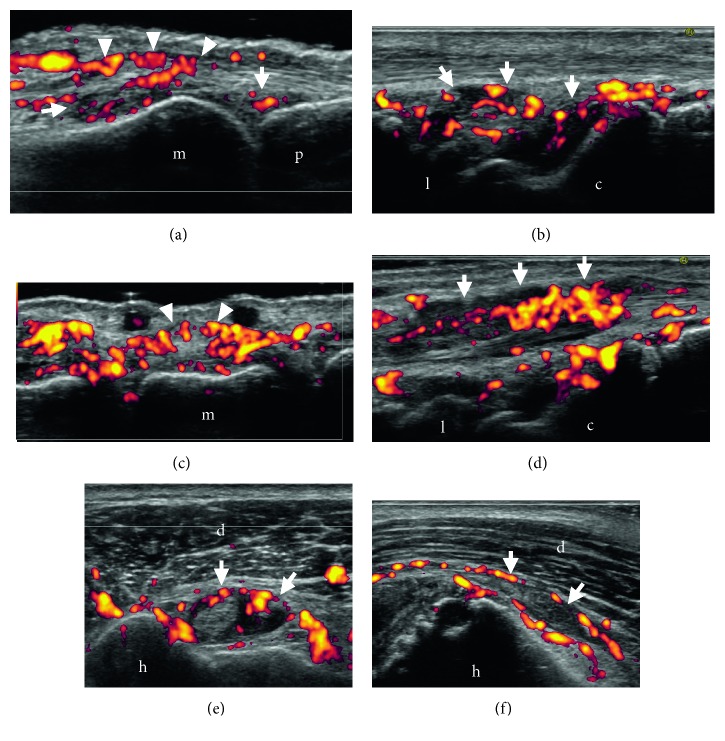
Severe synovitis detected by ultrasonography at 3 months after the introduction of nivolumab. (a) Articular synovitis (arrow) and peritendinitis of digital extensor tendon (arrowhead) in the 2nd metacarpophalangeal (MCP) joint (dorsal aspect, longitudinal view). (b) Articular synovitis (arrow) in a radiocarpal-intercarpal joint (dorsal aspect, longitudinal view). (c) Peritendinitis of digital extensor tendon (arrowhead) in MCP joint (dorsal aspect, transverse view). (d) Tenosynovitis (arrow) of the fourth compartment (extensor indicis and extensor digitorum communis) of the extensor tendons (dorsal aspect, longitudinal view). (e) Tenosynovitis (arrow) of the long head of the biceps (transverse view). (f) Subdeltoid bursitis (arrow) (longitudinal view). (c) Capitatum. (d) Deltoid muscle. (h) Humerus. (l) Lunatum. (m) Media. (p) Proximalis.
